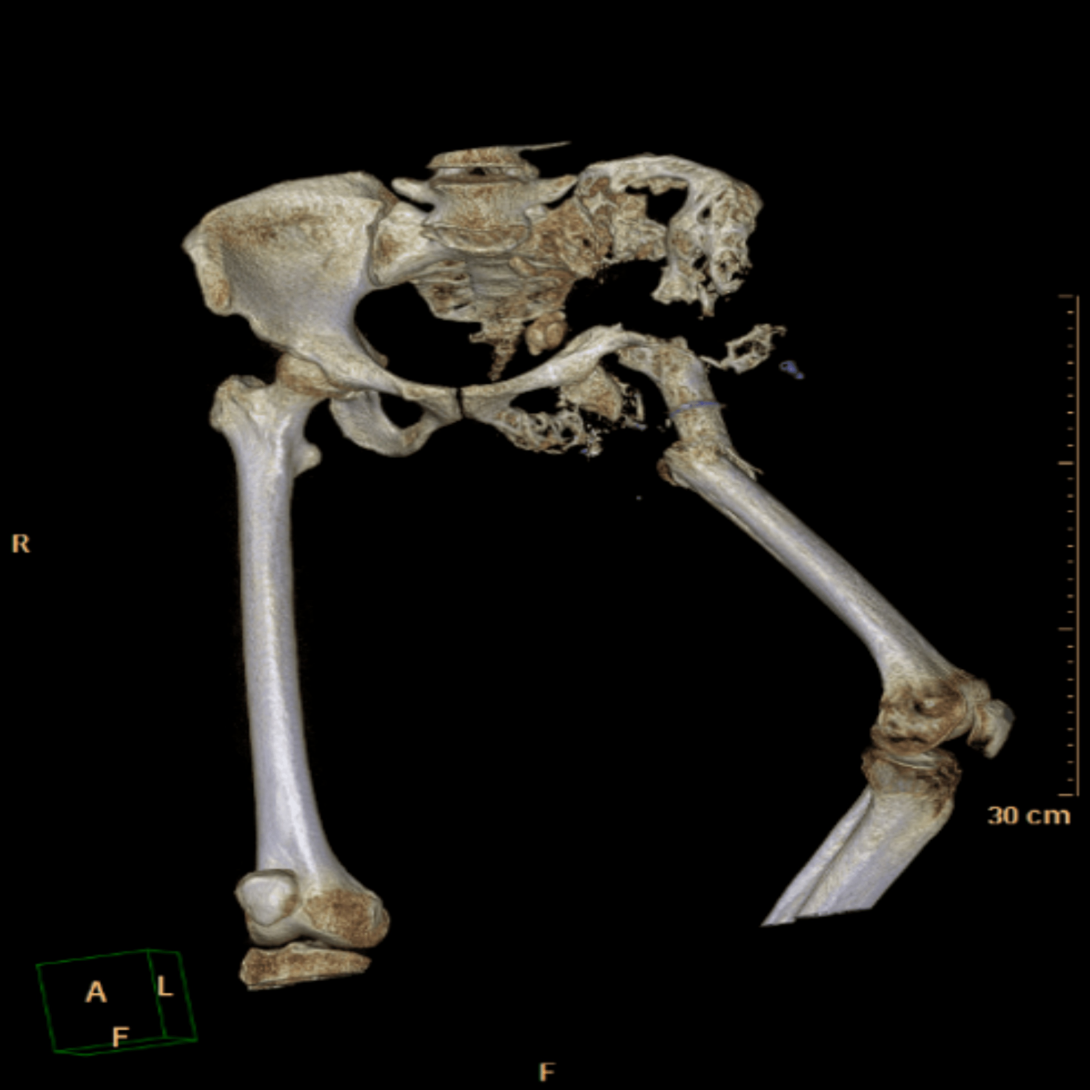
3D reconstructive image of the CT scan taken upon admission
